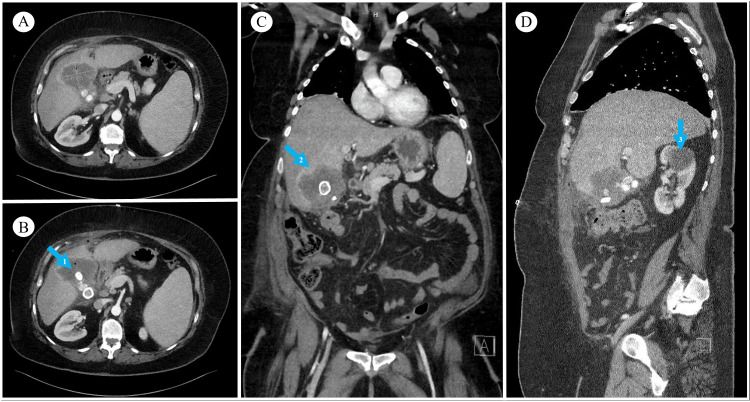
Inpatient CT chest/abdomen/pelvis with IV contrast in axial (A, B), coronal (C), and sagittal views (D). CT images reveal gallstones (arrow 1) and irregular thickening of the gallbladder wall with enhancement and pericholecystic infiltration (arrow 2). Air is present within the gallbladder as well as anterior to the liver. These findings indicate gallbladder carcinoma with hepatic invasion, and cholelithiasis. There is a right renal cyst present (arrow 3), which was incidentally discovered.
